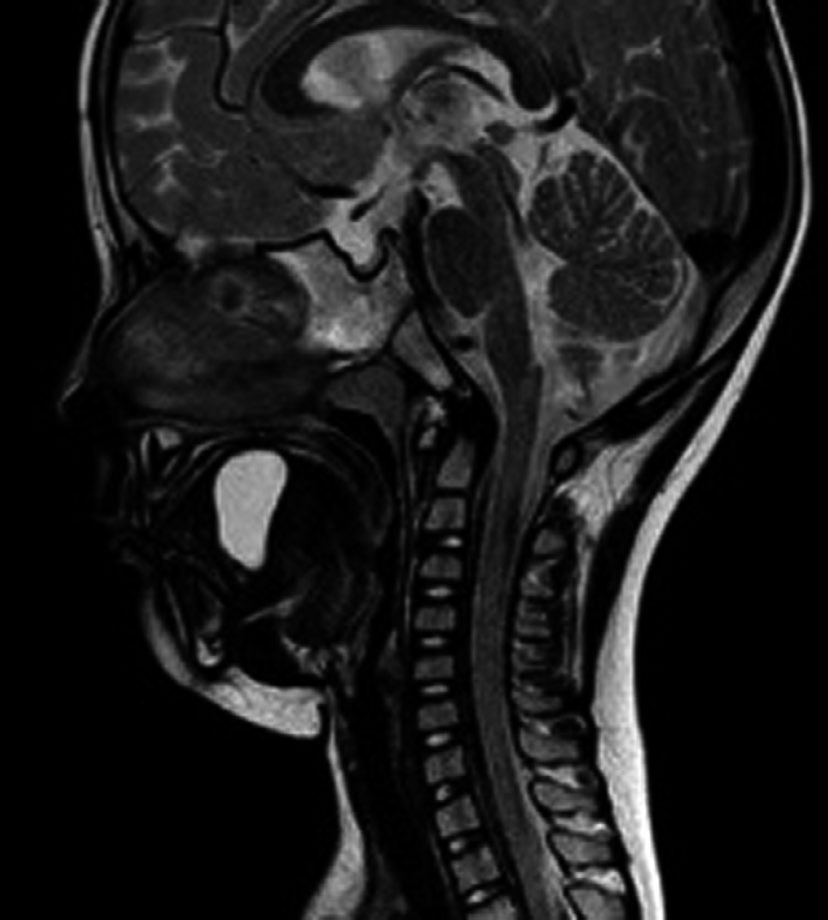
Magnetic resonance images. Sagittal section/a cystic lesion with clear margins is observed
